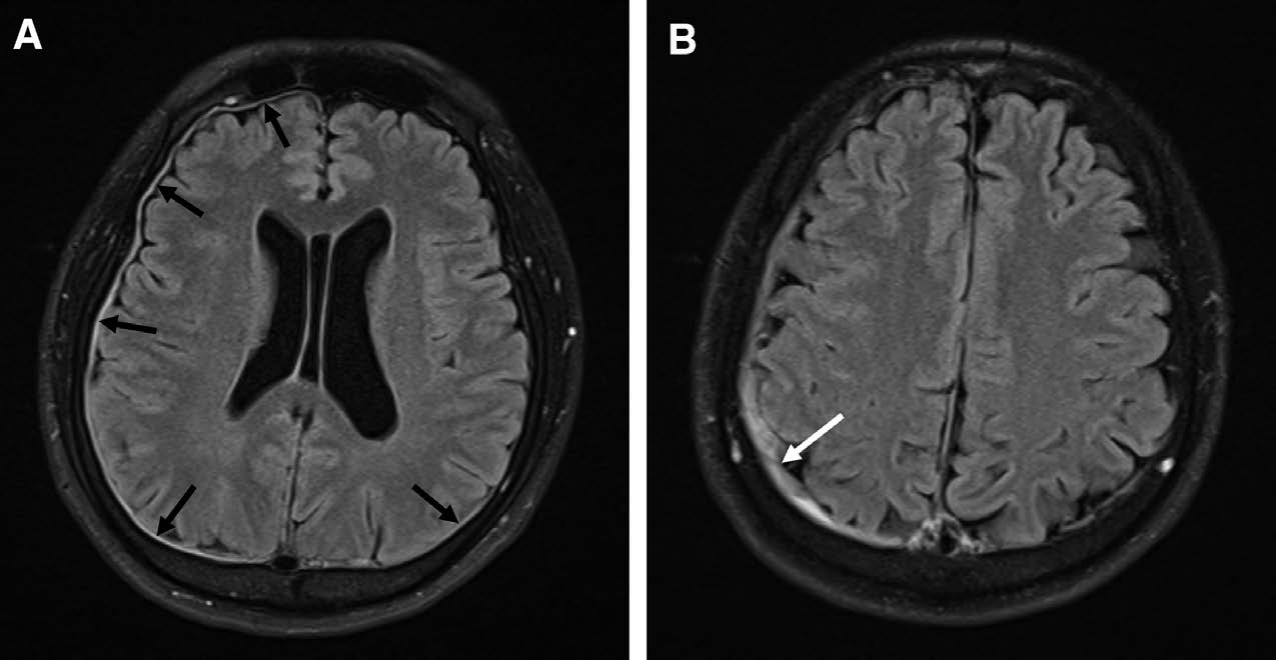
MRI images showing pachymeningeal enhancement and SDH. Contrast-enhanced T1-weighted MRI images shows diffuse pachymeningeal enhancement (A, black arrows), which is a radiological finding characteristic of SIH, and a small SDH (B, white arrow). MRI = magnetic resonance imaging, SIH = spontaneous intracranial hypotension, SDH = subdural hematoma.
